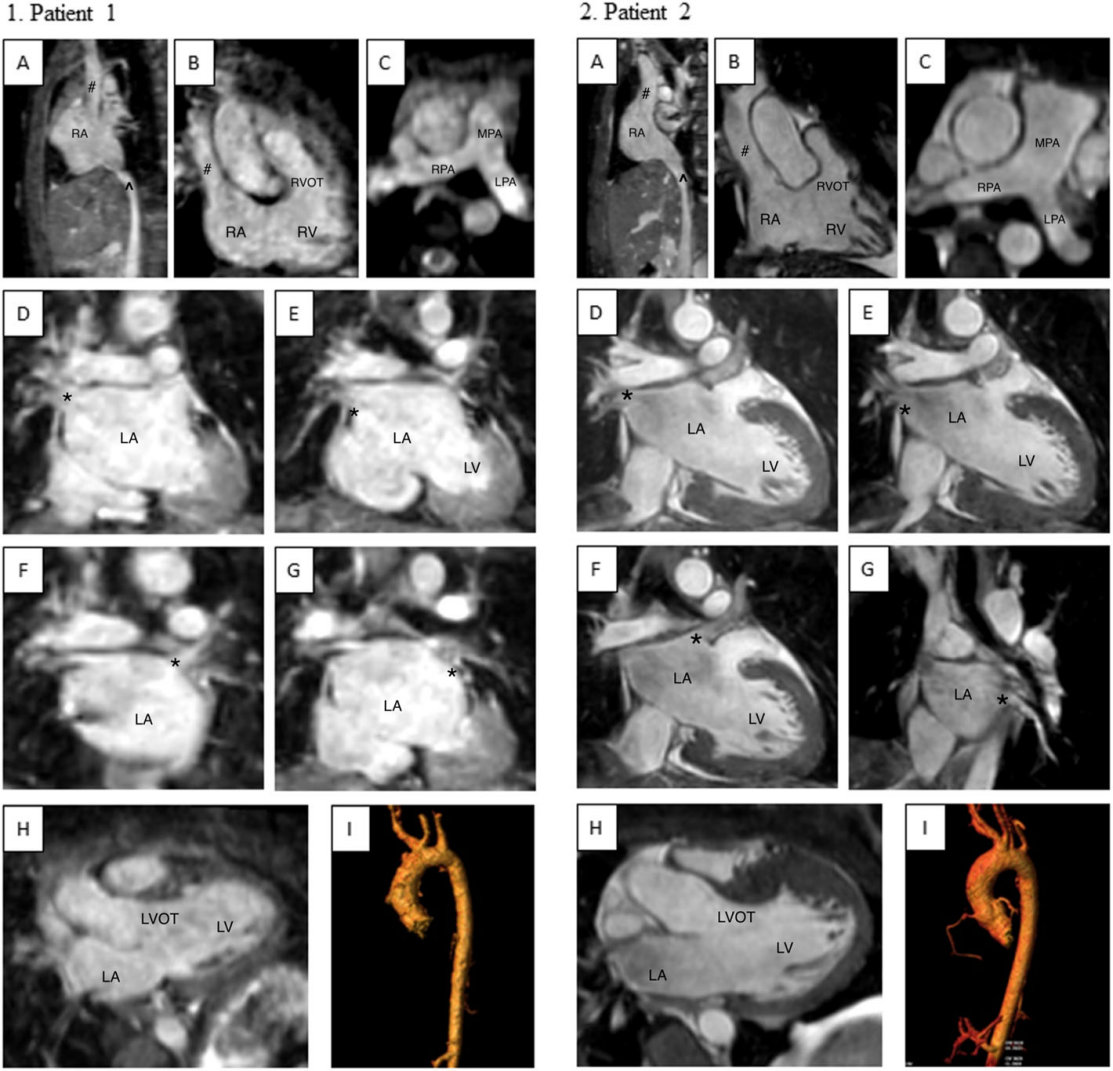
Representative 3D WH bSSFP images from two patients obtained with iNAV. This figure shows the range of image quality that still allowed complete morphological diagnosis (ie. all structures identified without severe blurring [18]) 1. Collection of pictures from one patient with lower quality. Patient presented with chest pain and bicuspid aortic on echocardiogram with a suspected abnormal left coronary artery origin. Complete morphological diagnosis was possible; however, this collection is a representative of patients with slightly lower quality. 1A. SVC and IVC enter into the RA normally. 1B. SVC entering RA normally, RA to RV connection with RVOT visualized. 1C. Normal branch pulmonary arteries. 1D. Right upper pulmonary vein (*) entering into the LA. 1E. Right lower pulmonary vein (*) entering the LA. 1F. Left upper pulmonary vein (*) entering the LA. 1G. Left lower pulmonary vein (*) entering the LA. 1H. LA to LV connection with LVOT visualized. 1I. Ascending aorta, arch, head and neck vessels and descending aorta. The left carotid artery arises from the trunk of the innominate artery. 2. Higher quality pictures from another patient with hypertrophic cardiomyopathy. Complete morphological diagnosis was also possible in this patient. 2A. SVC and IVC enter into the RA normally. 2B. SVC entering RA normally, RA to RV connection with RVOT visualized. 2C. Normal branch pulmonary arteries. 2D. Right upper pulmonary vein (*) entering the LA. 2E. Right lower pulmonary vein (*) entering the LA. 2F. Left upper pulmonary vein (*) entering the LA. 2G. Left lower pulmonary vein (*) entering the LA. 2H. LA to LV connection with LVOT visualized. 2I. Ascending aorta, aortic arch, head and neck vessels and descending aorta. The left carotid artery and innominate artery arise from a common trunk from the arch. There is also a vertebral artery arising directly from the arch. iNAV = image navigator; SVC = superior vena cava (#); IVC = inferior vena cava (^); RA = right atrium; RV = right ventricle; RVOT = right ventricular outflow tract; MPA = main pulmonary artery; RPA = right pulmonary artery; LPA = left pulmonary artery; pulmonary veins labeled with asterisk (*); LA = left atrium; LV = left ventricle; LVOT = left ventricular outflow tract
